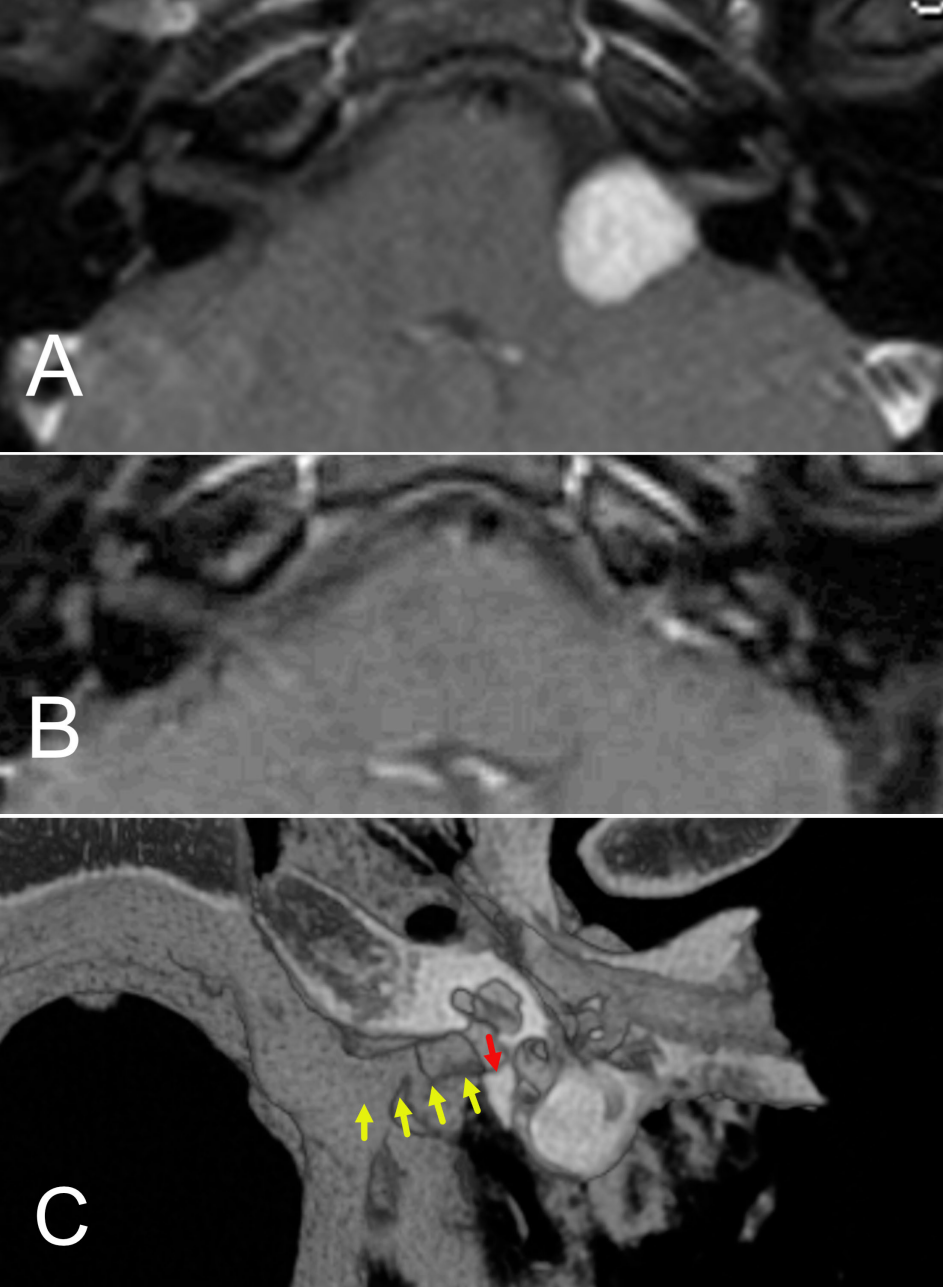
(A, B) Preoperative (A) and postoperative (B) MRI of a patient who underwent retrolabyrinthine hearing preservation of an acoustic neuroma. (C) 3D reconstruction of post operative CT scan for the patient operated by the retrolabyrinthine approach. The yellow arrows show the degree of internal auditory canal bone removal. The red arrow shows the final portion of bone that remained. At least 4/5 of the length of the internal canal can be removed and the inner ear structures retained.
